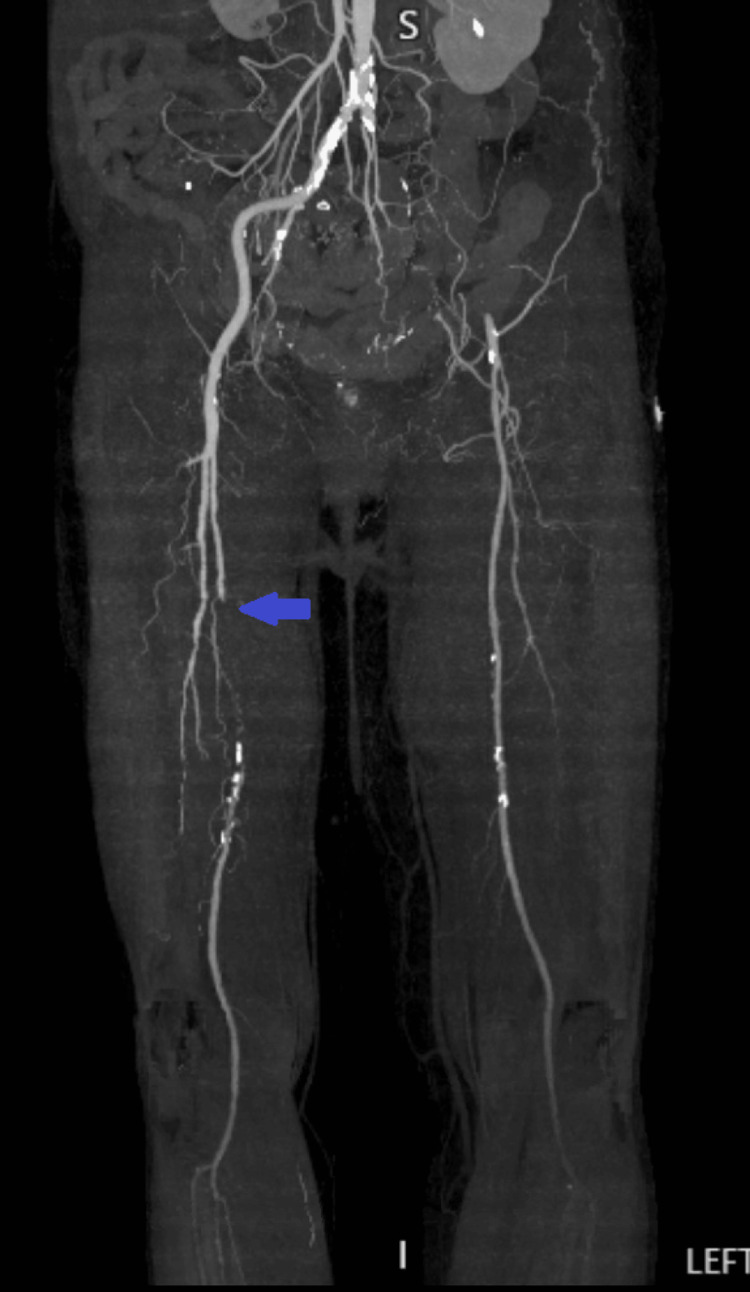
CT angiography imaging demonstrating occlusion of the right superficial femoral artery at the level of the arrow
